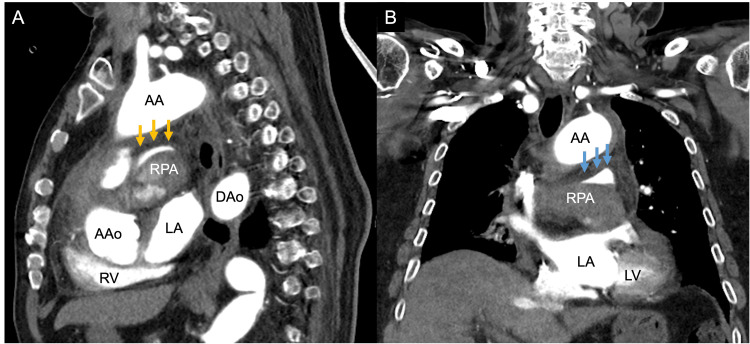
Contrast-enhanced computed tomography of the right pulmonary artery main trunk On sagittal view (A), the right pulmonary artery is compressed from below, resulting in a crescent-shaped lumen (yellow arrow). On coronal view (B), tapering stenosis extends from the main pulmonary artery (blue arrow). AA: aortic arch; AAo: ascending aorta; DAo: descending aorta; LPA: left pulmonary artery; LPV: left pulmonary vein; MPA: main pulmonary artery; RPA: right pulmonary artery
